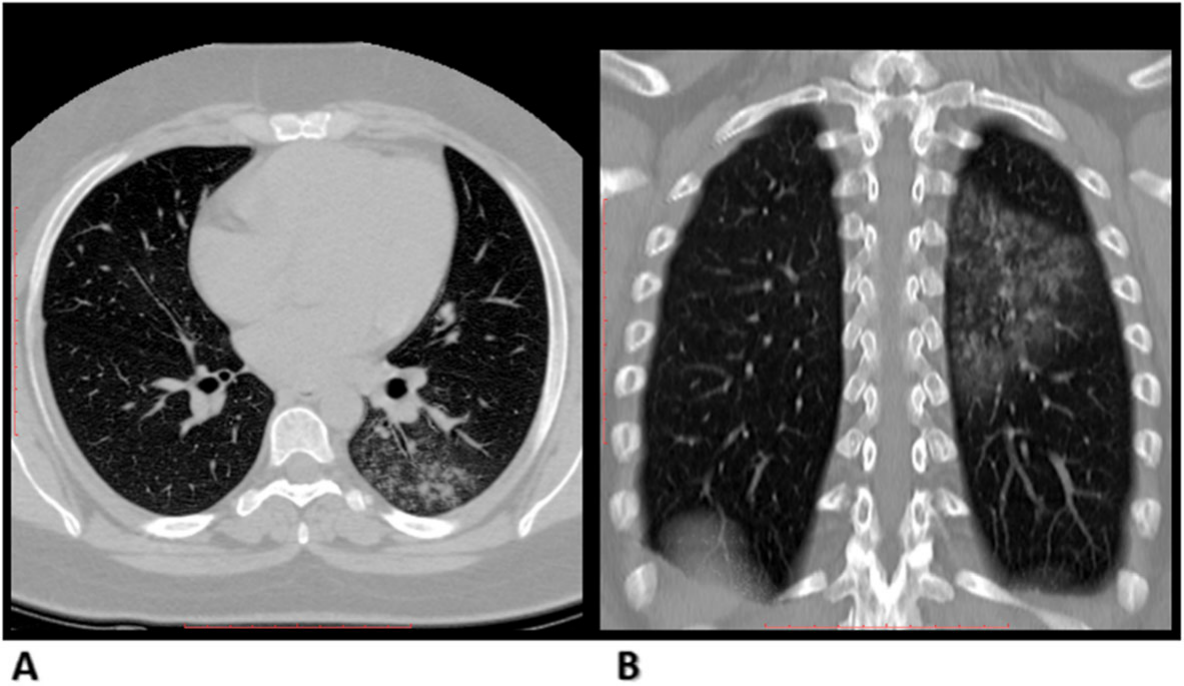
A 23-year-old woman with CT findings of consolidation in the left lower lung lobe with the tree on bud appearance Observers agreed on COVID-RADS score 1 and CO-RADS score 2. The preference of COVID-RADS: CO-RADS was (1:2). RT-PCR result was negative
